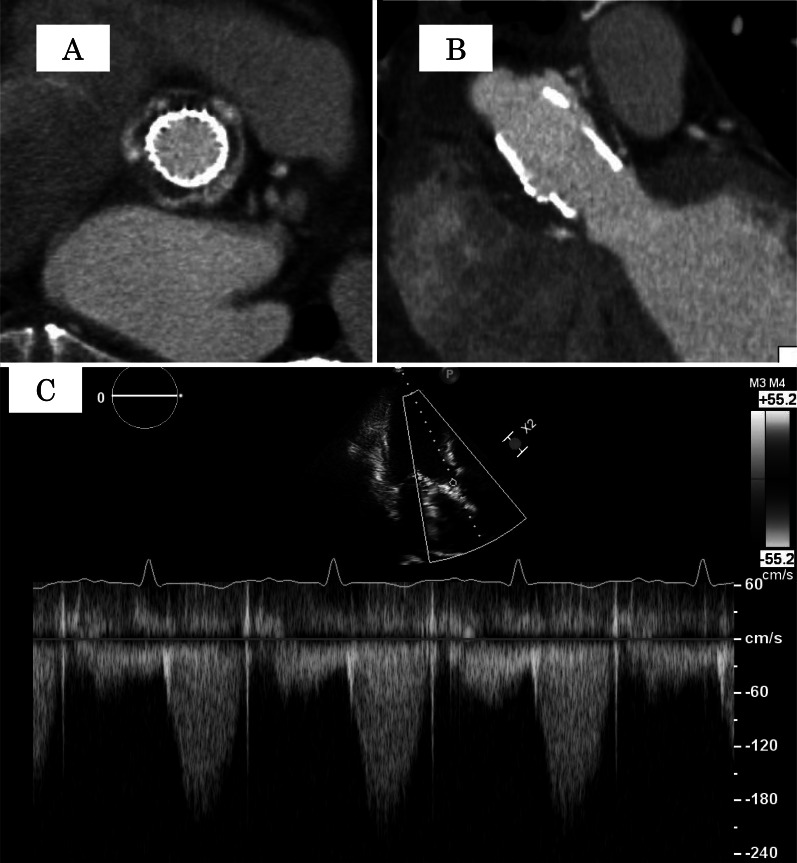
Postoperative CT scan and echocardiography. A postoperative CT scan demonstrates that the sutureless valve adequately expanded at the annulus level (A short axis view, B long axis view). The pressure gradient through the valve was ten mmHg, and no paravalvular leakage was observed (C)
